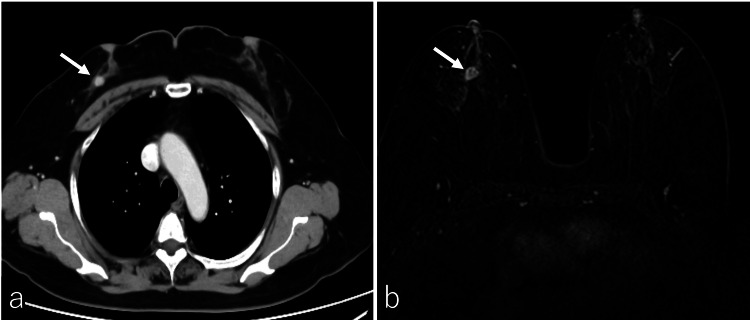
Computed Tomography and Magnetic Resonance Imaging Computed tomography (CT) revealed a 13 mm nodule in the lower lateral aspect of the right breast with no apparent metastatic lesions (a, arrow). Magnetic resonance imaging (MRI) showed a 12 mm nodule in the right breast, consistent with the CT findings (b, arrow).
